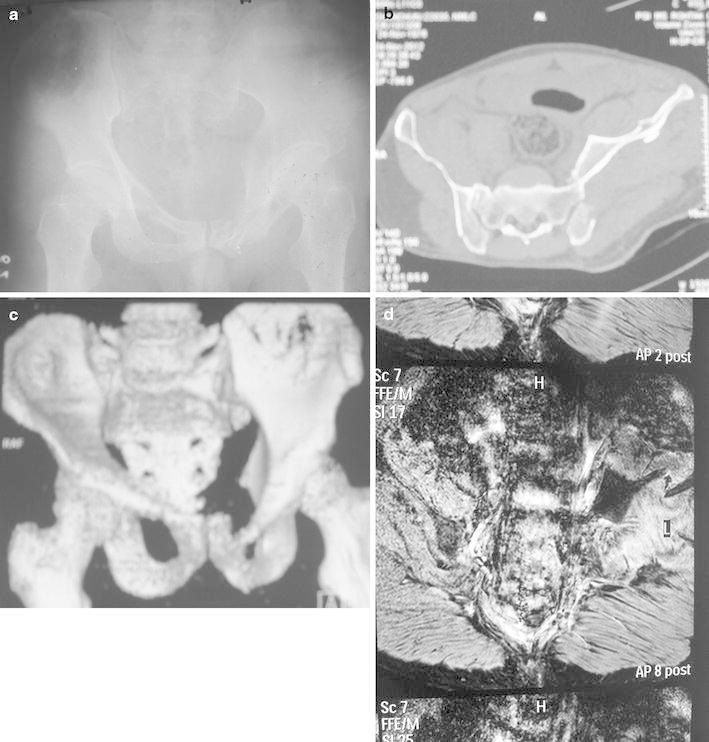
a Radiograph showing the sacroiliac fracture dislocation with fractures of bilateral pubic rami. b A CT scan (transverse section) showing the anterior dislocation of ilium over sacrum. c A CT scan (3D) showing fracture pubic rami with anterior displacement of iliac crest along with part of sacrum. d An MRI scan showing integrity of lumbosacral plexus
